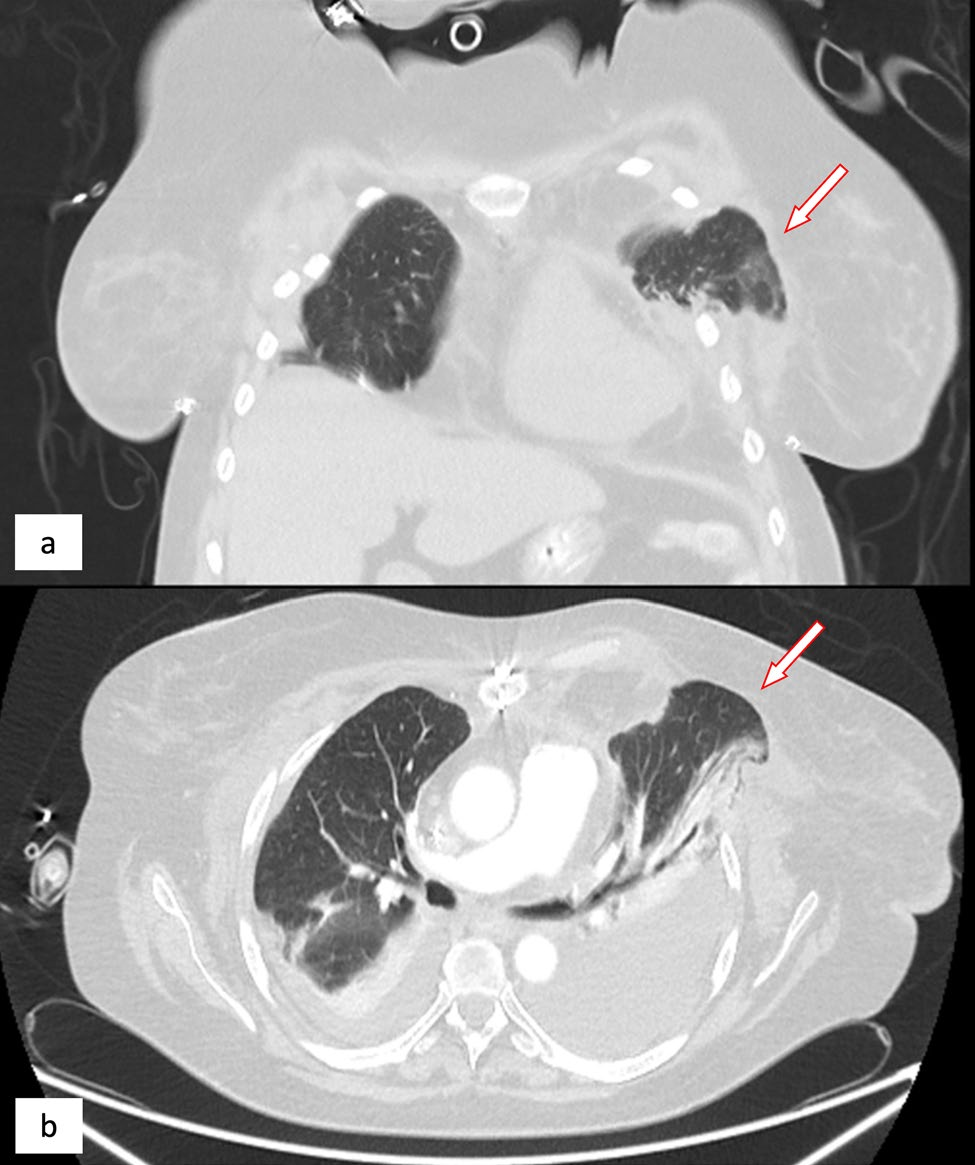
Chest CT scan, coronary (a) and transversal (b) axis. Arrows highlighting left lung herniation at 4th intercostal space
